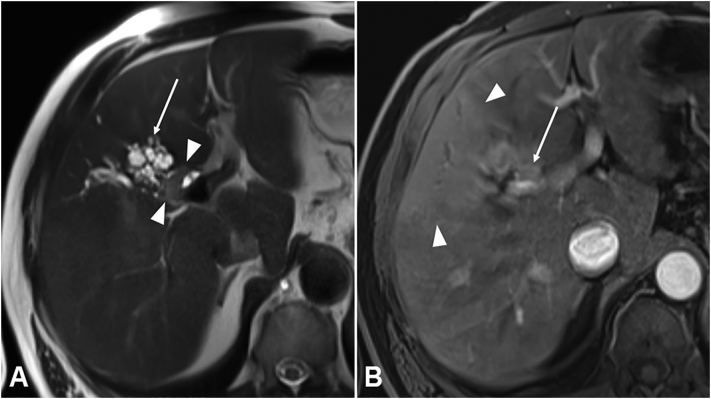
(A) Axial T2 weighted image of follow up MRI with MRCP showing the cystic lesion in liver segment V with a new soft tissue component of 1,7 cm (arrowheads) adjacent to the right hepatic duct. (B) Axial T1 weighted fat suppressed image in the arterial phase after intravenous administration of a gadolinium based contrast agent (gadoxetate disodium, Primovist ™, Bayer Healthcare Pharmaceuticals) demonstrating altered perfusion of liver segment V (arrowheads), dilated bile ducts in liver segment V and VIII and moderate compression of the middle portal vein (trifurcation of the portal vein as anatomic variant) due to the soft tissue lesion in Segment V (arrow).
